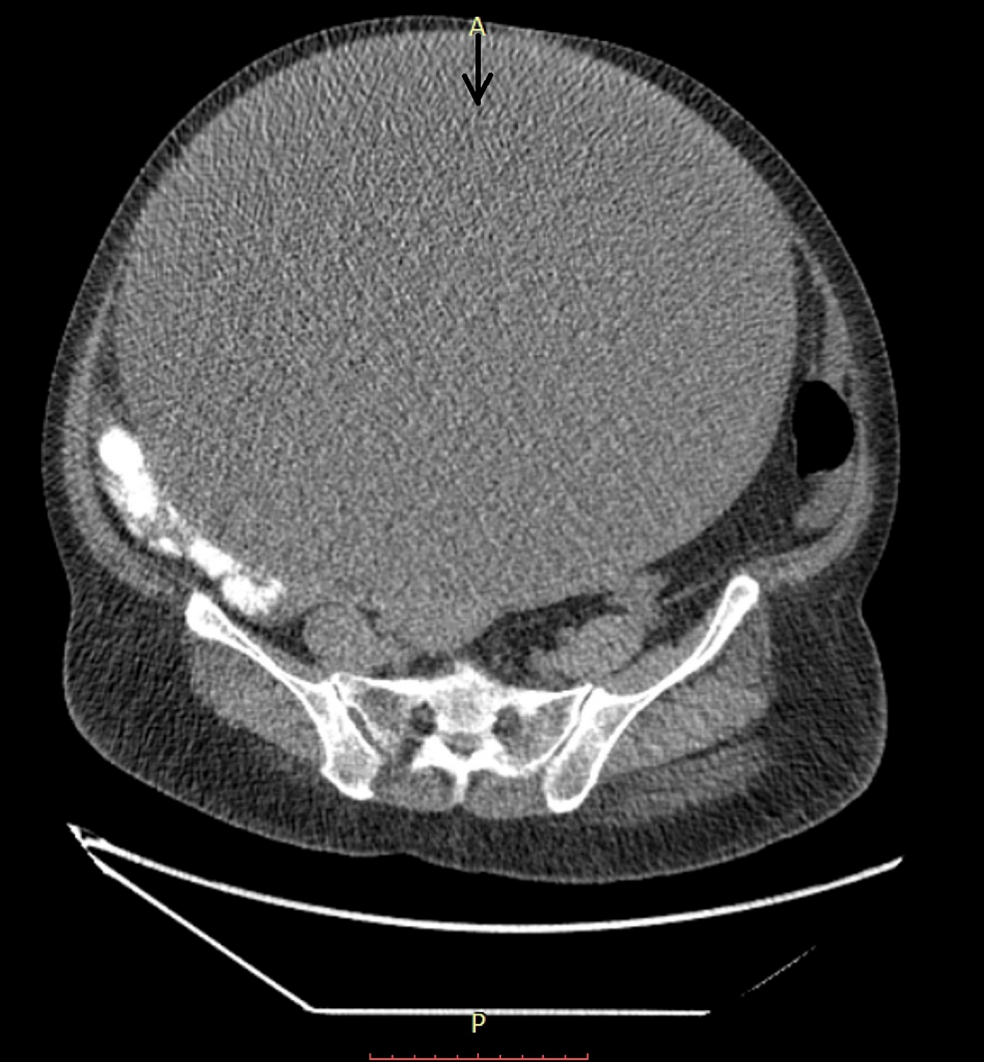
Abdominal CT scan showing a giant ovarian cyst The black arrow shows the ovarian cyst. A: anterior side; P: posterior side; CT: computed tomography.
